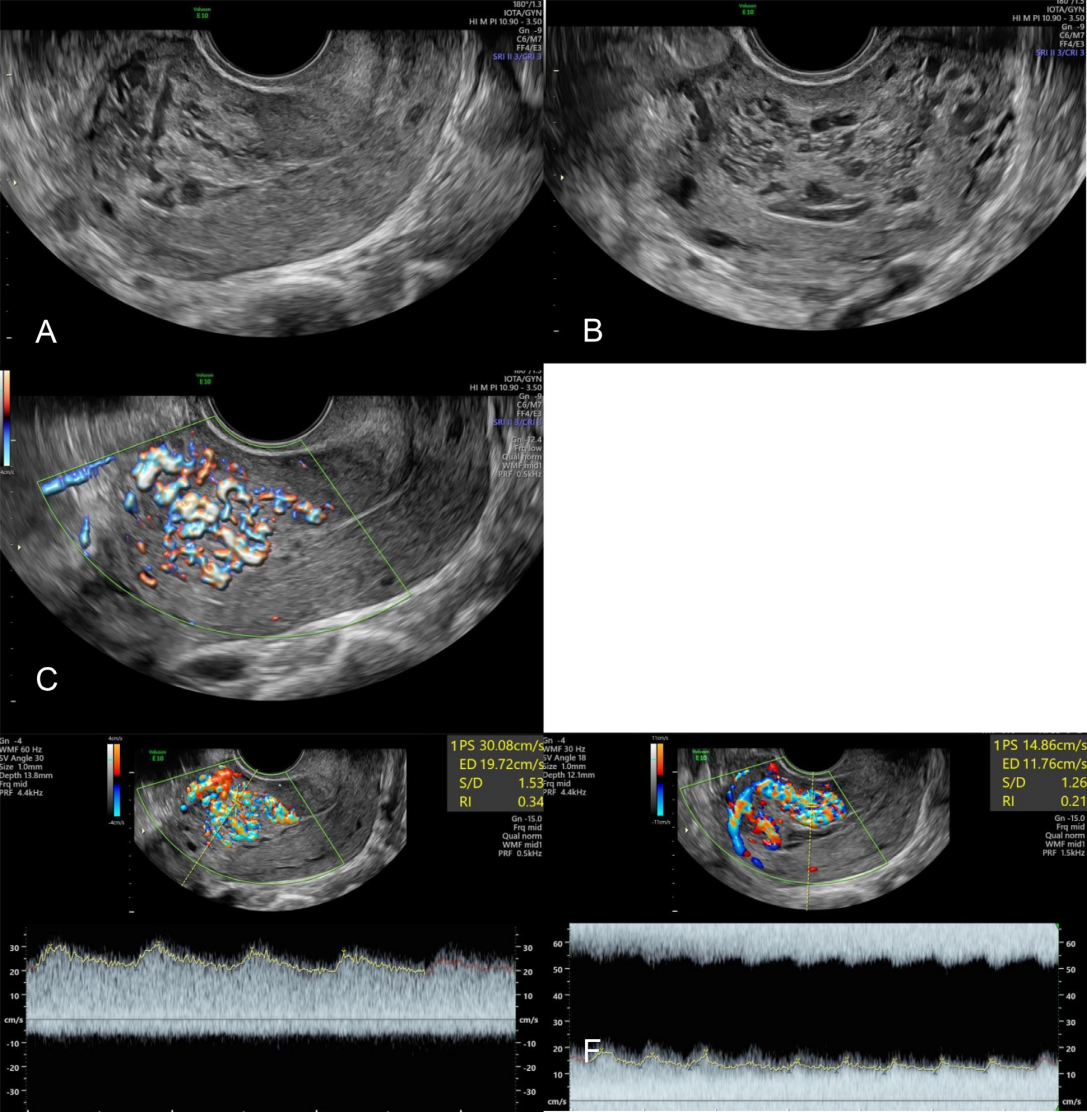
From top left showing heterogeneous tissue between the endometrium in (A) longitudinal view and (B) transverse view. (C and D) with color Doppler showing vascularity in the anterior wall of the uterus in longitudinal and transverse views, and lastly (E) and (F) showing Doppler flow with resistance indices (RI) of 0.34 and 0.21.
